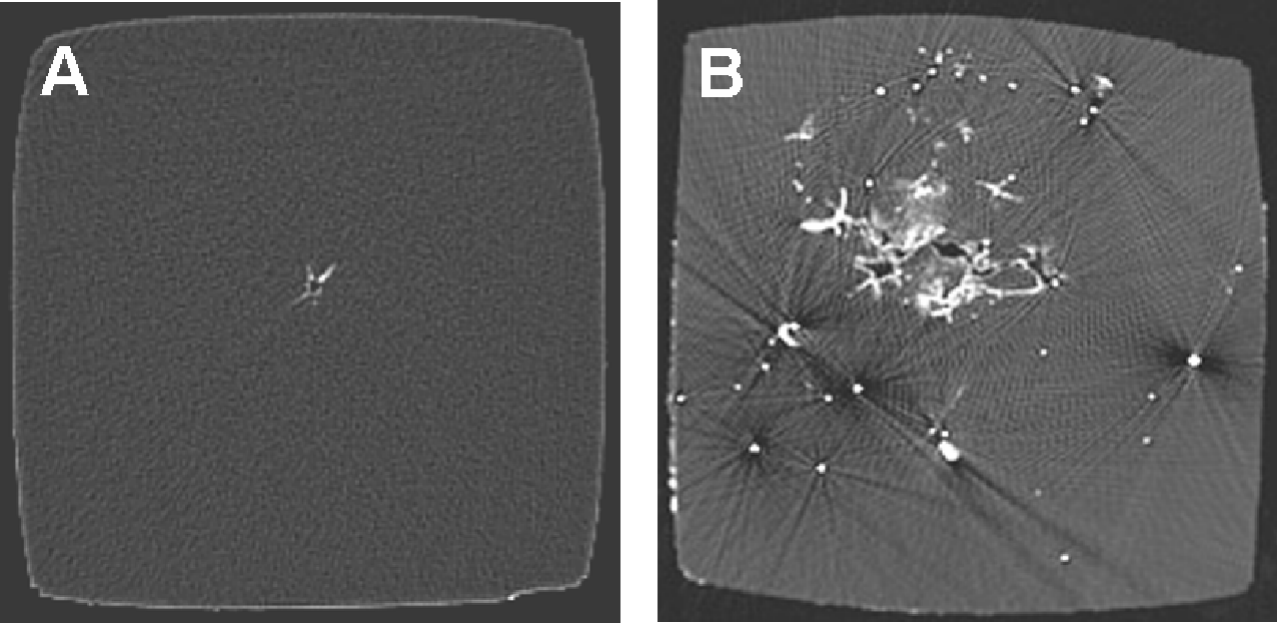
CT-scans of vertical section through the gelatin block 8 cm from the bullets point of entry. While in the full metal jacket bullets path (A) only a small permanent cavity with little barium titanate was detectable, the soft point projectile fragmented and lead to a completely different wound characteristics (B).
